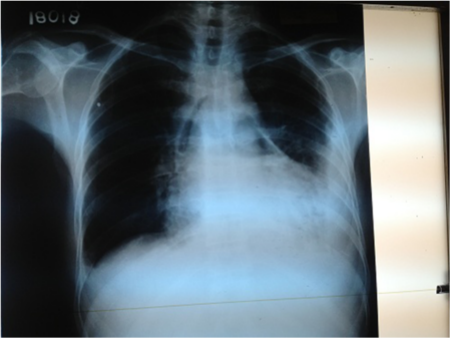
Chest X-Ray – Chest X-Ray – Anterior-Posterior View of the patient showing air and fluid around the heart.
